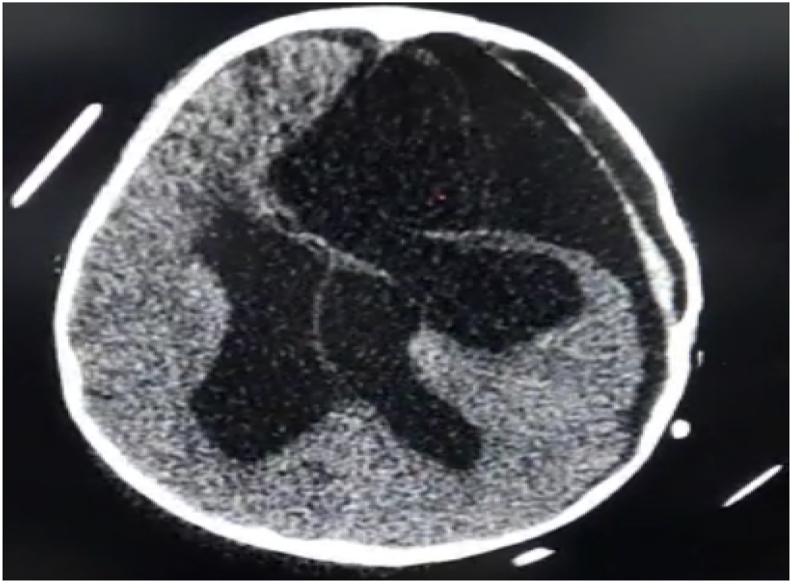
The control CT scan showing the total removal of the hydatid cyst in the second patient.
